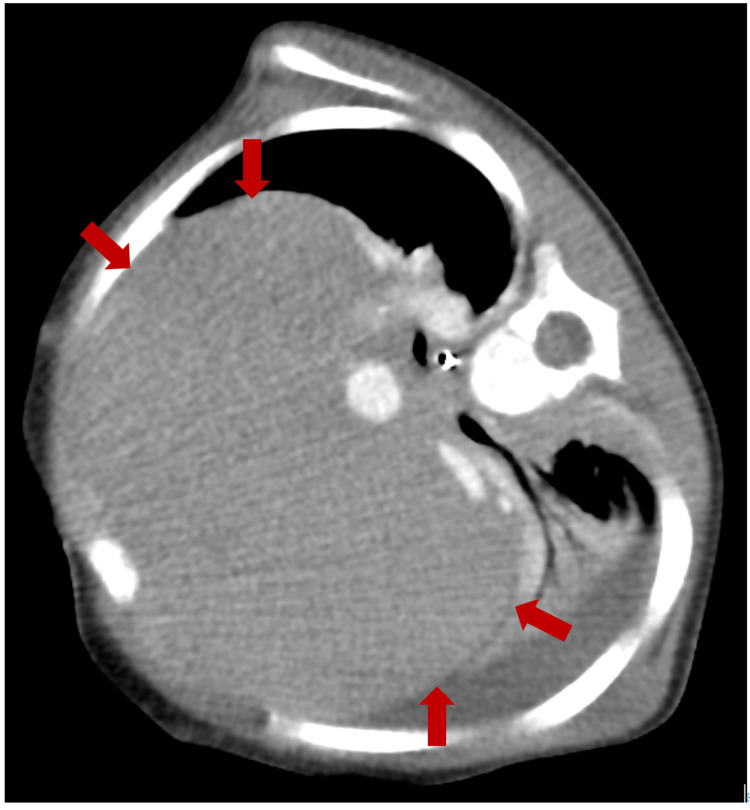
Contrast-enhanced CT of chest Contrast-enhanced CT of the chest reveals an anterior mediastinal tumor measuring 114×74×97 cm.
